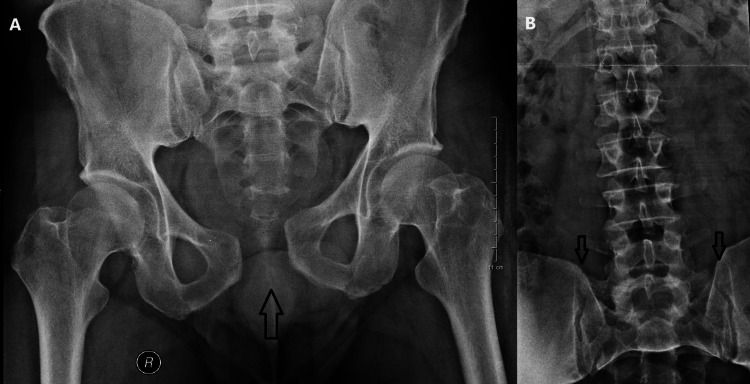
A) Initial anteroposterior radiograph of pelvis displaying wide diastasis of the pubic symphysis. B) Anteroposterior radiograph of the lumbar spine depicting no sign of sacroiliac joint disruption.
